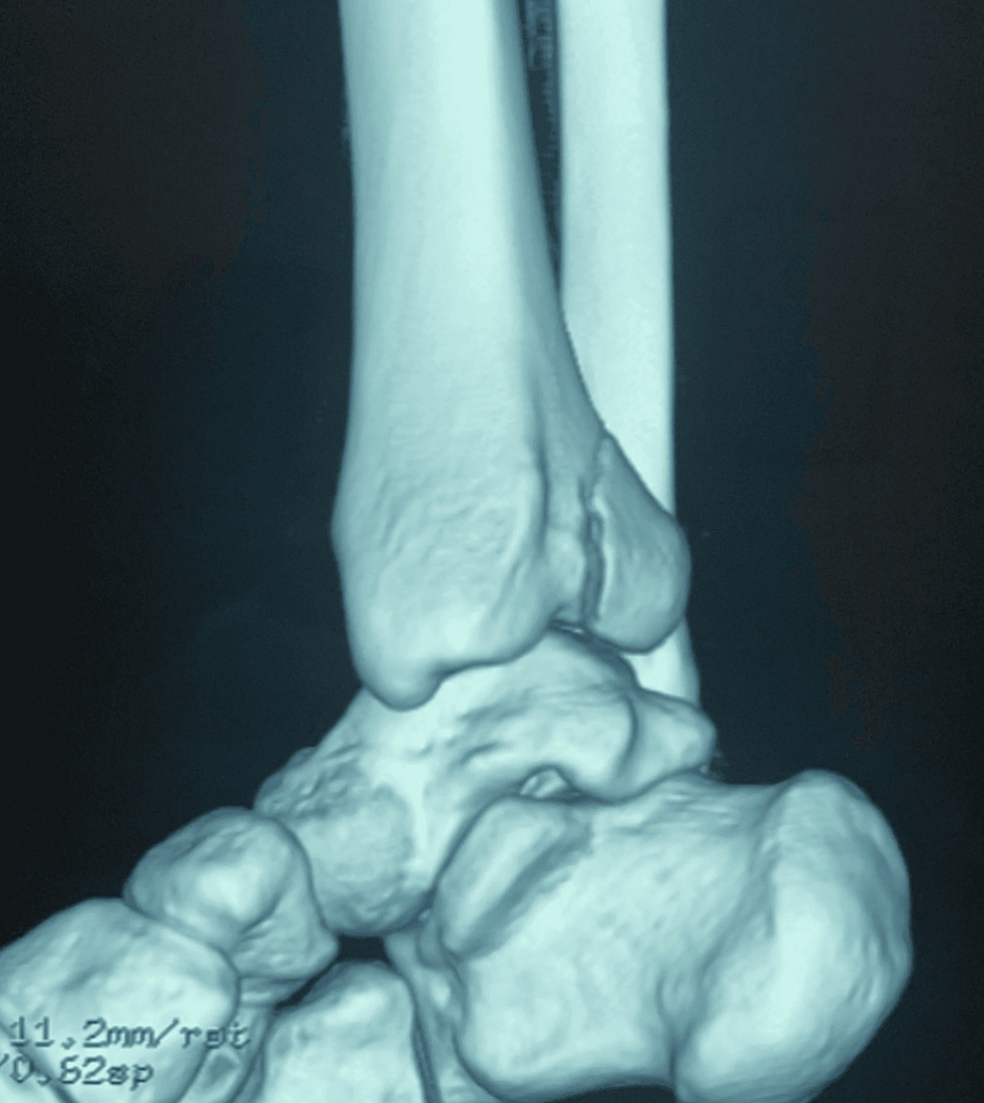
CT scan with a 3D reconstruction of the ankle showing the isolated fracture of posterior tibial margin
